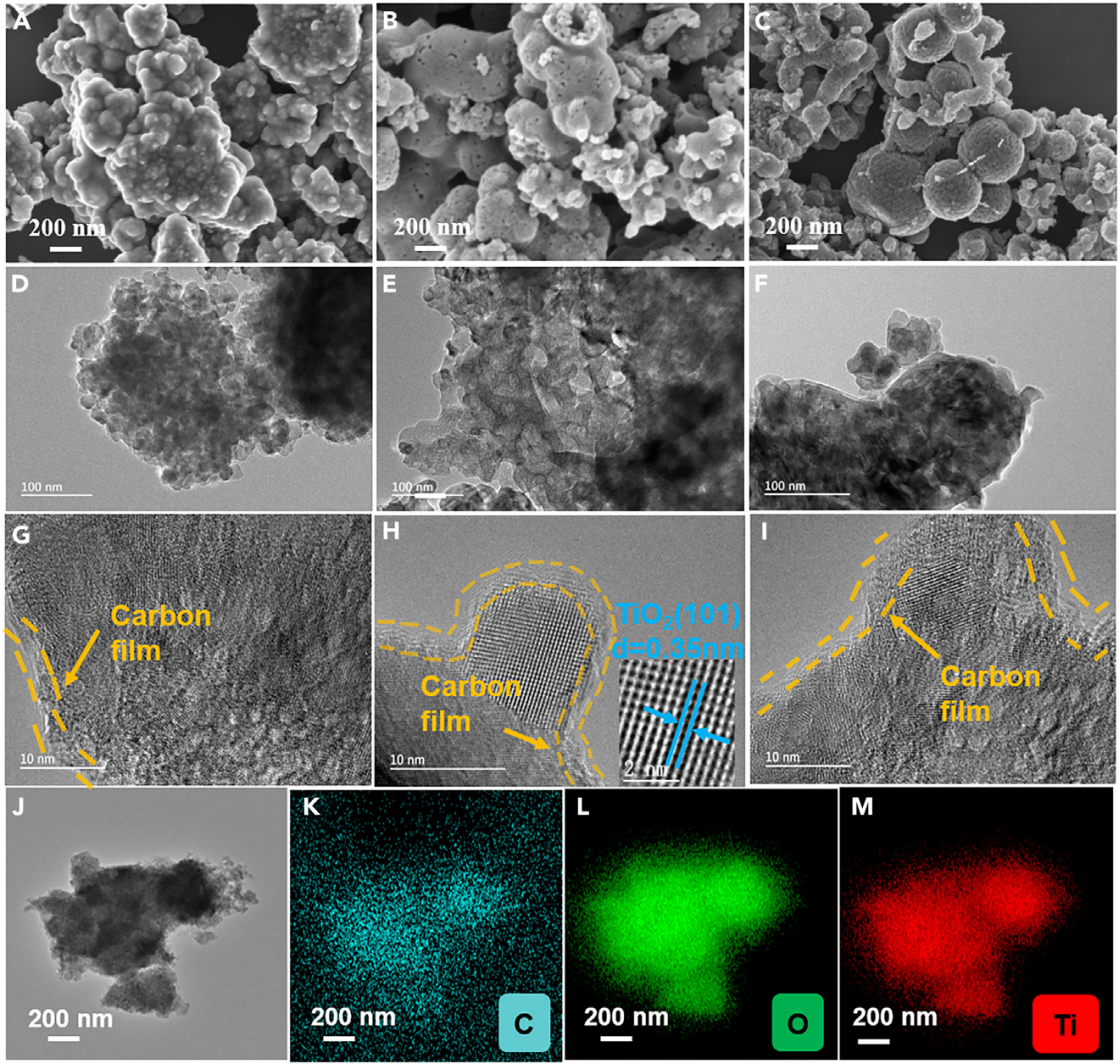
Atomic-resolution structure characterization and EDS patterns of the V_O_-CT, V_O_-MCT, V_O_-SCT (A–C) SEM images of (A) V_O_-CT, (B) V_O_-MCT, (C) V_O_-SCT. (D–F) TEM images of (D) V_O_-CT, (E) V_O_-MCT, (F) V_O_-SCT. (G–I) HRTEM images of (G) V_O_-CT, (H) V_O_-MCT, (I) V_O_-SCT. (J–M) EDS elemental mappings of V_O_-MCT. See also Figure S3.
